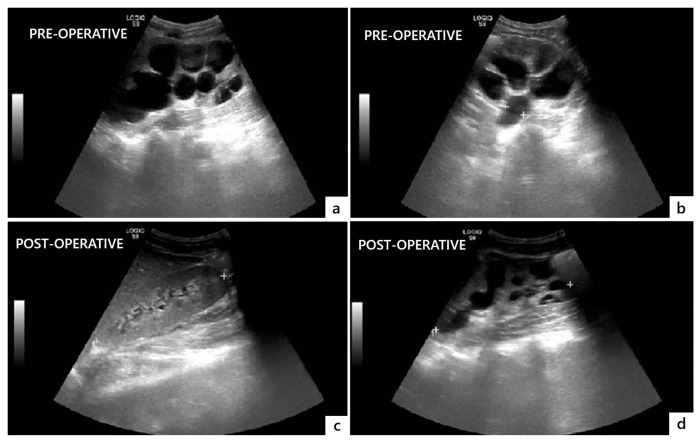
Comparison of preoperative (a,b) and postoperative (c,d) ultrasonographic findings showing resolution of bilateral hydronephrosis and megaureter three months after surgery.
